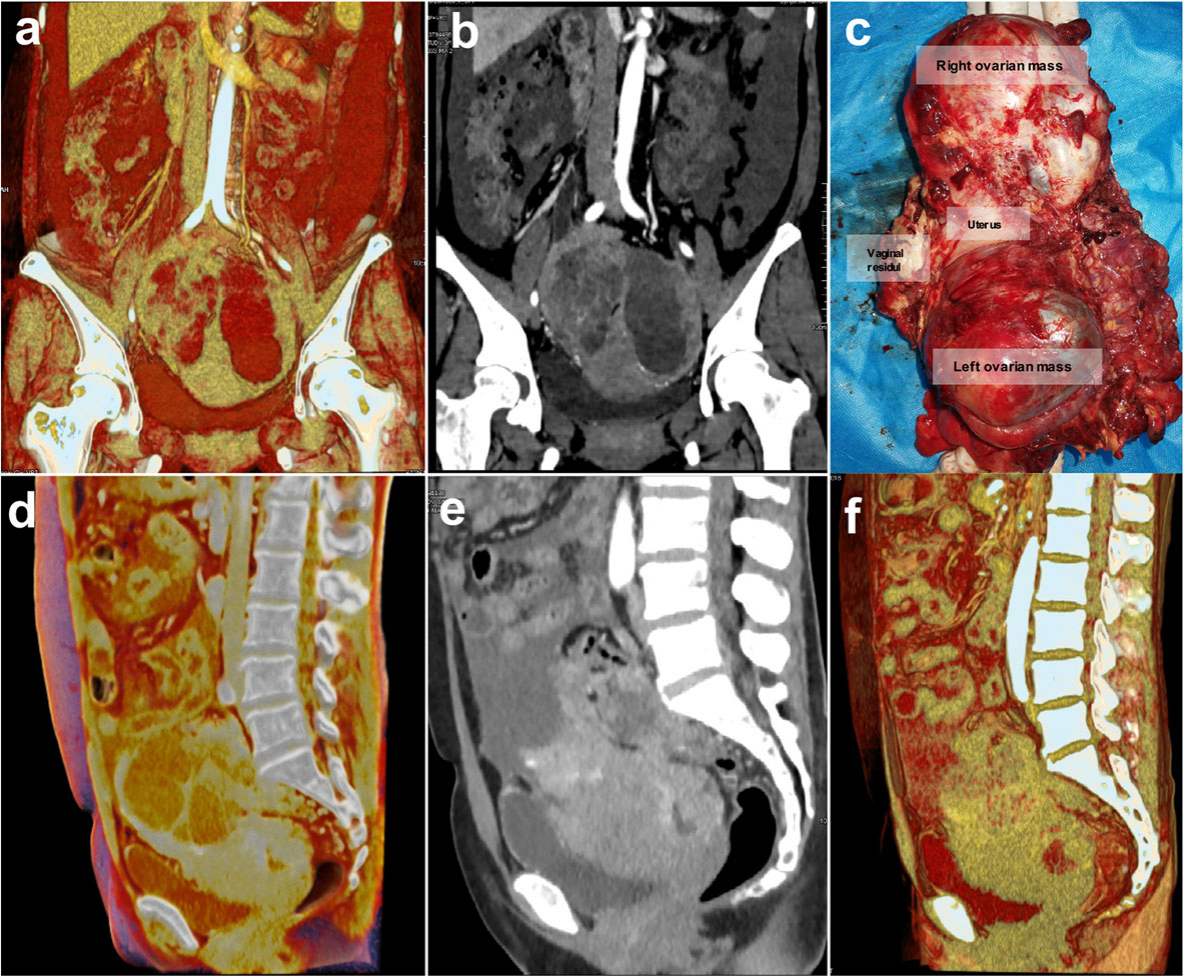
The adhesion of the pelvic mass to the rectum and bladder in CT images and gross pathology (case 2). (a-b) The large ovarian mass closed the entrance to the pelvis. The lesion was a mixed type of cyst, which was closely connected with the iliac vessels and lateral wall of the pelvic cavity. (c) There was no distinct boundary between the mass and the uterus located in the rear. (a, d) The coronal and sagittal CR image showed that the ovarian mass violated the posterior wall of the bladder and the anterior rectal wall. The normal muscle wall structure of the above cavity viscera disappeared completely. (b, e) In the MPR image, the boundary of the lesion was displayed because of the ascites and urinary bladder, but the depth of the organ invasion was still difficult to determine. (f) VR showed poor effect and could hardly distinguish the lesion from its adjacent organs
